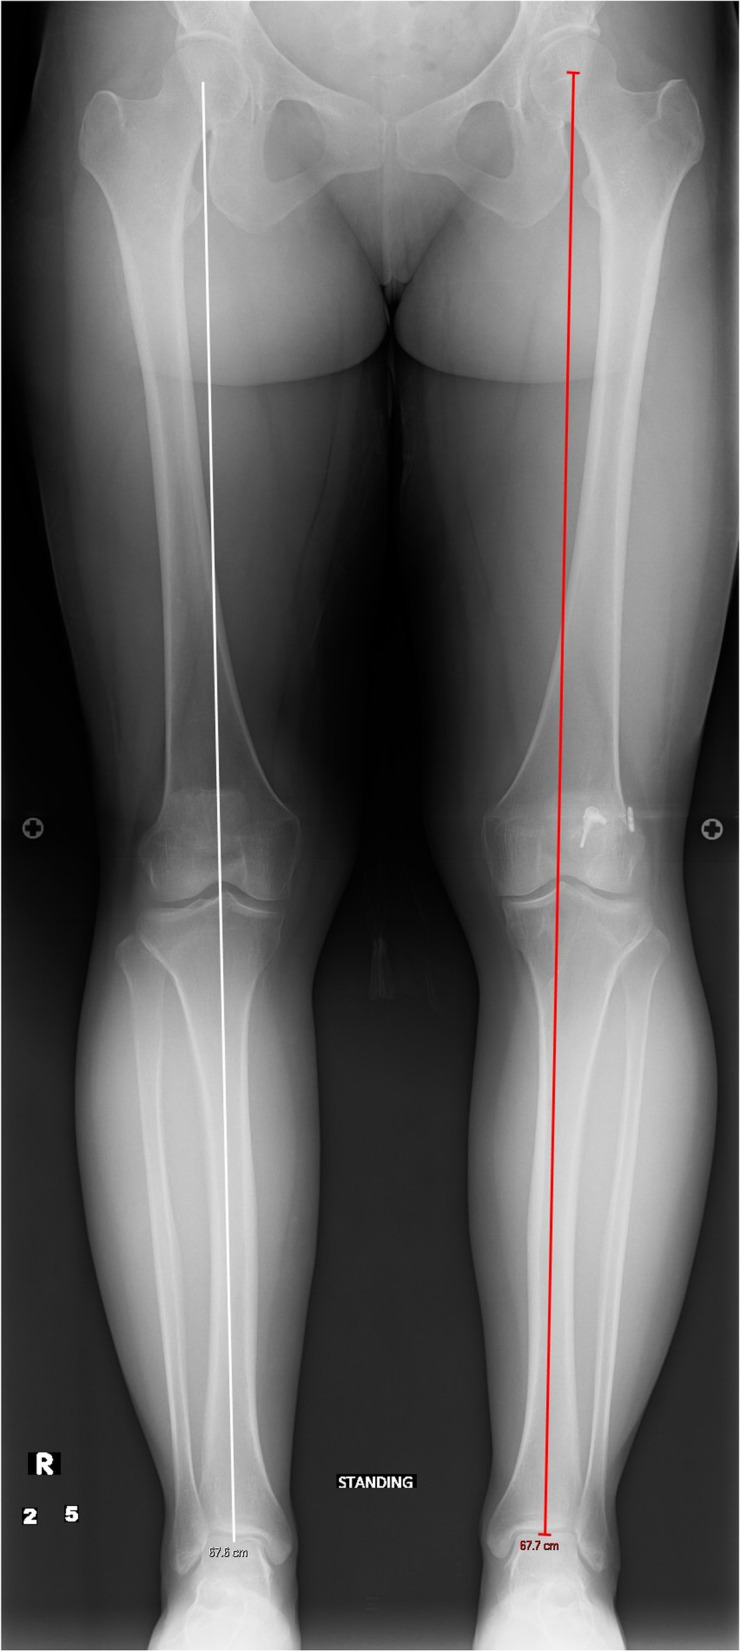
A pre-operative standing mechanical axis radiograph demonstrating physiologic varus
